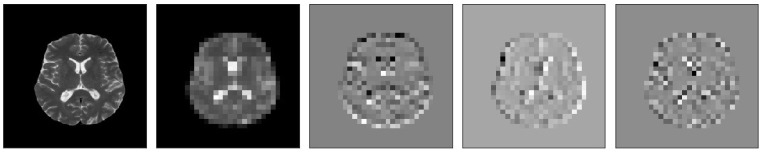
Samples of our Level 3 DWT decomposition of a healthy MR image type T2.
